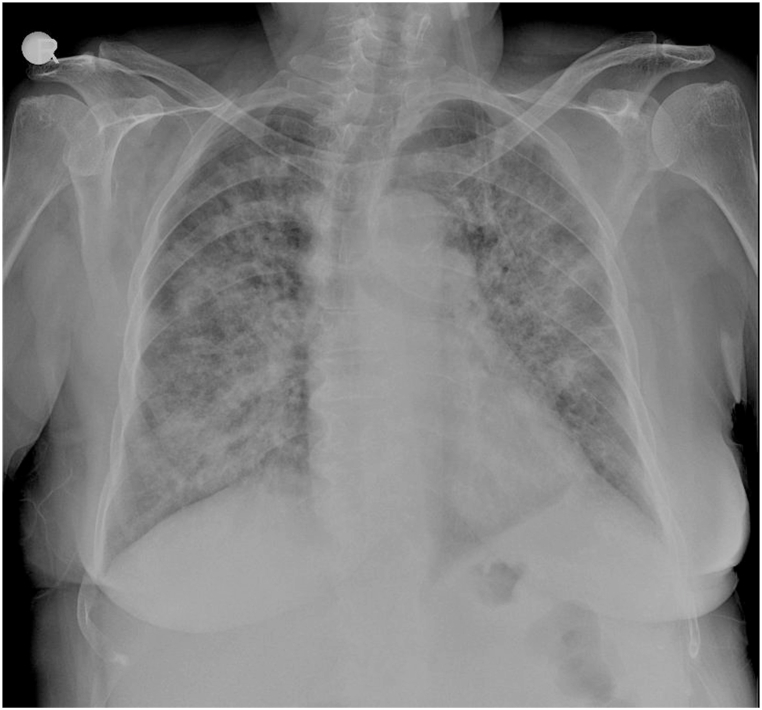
An X-ray film shows ground-glass opacities bilaterally.
